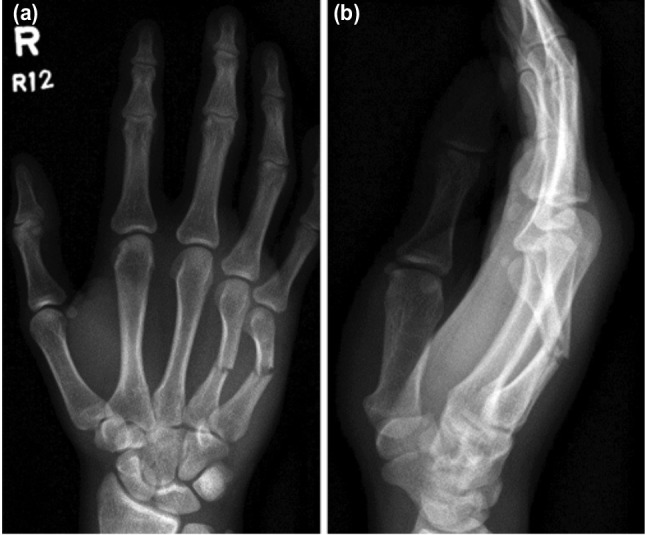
Pre-operative radiographs of (a) PA and (b) Lateral views of transverse mid metacarpal ring and little finger shaft fractures.
